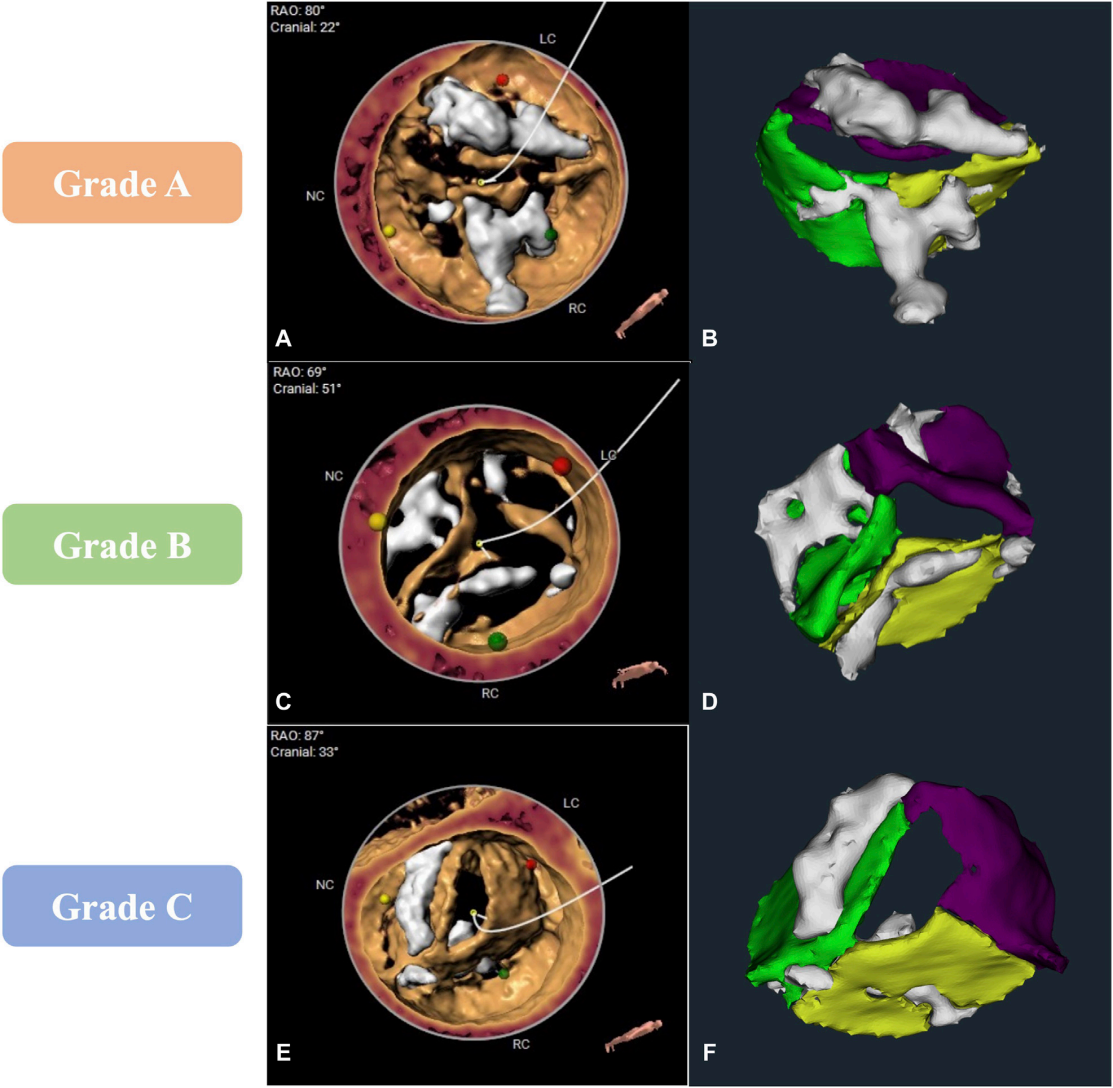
Comparison of computed tomography angiography image analysis results between 3mensio and the CVPILOT system. (A,B), (C,D), and (E,F) represent images reconstructed by the CVPILOT system that reached grade A, B, and C, respectively.
